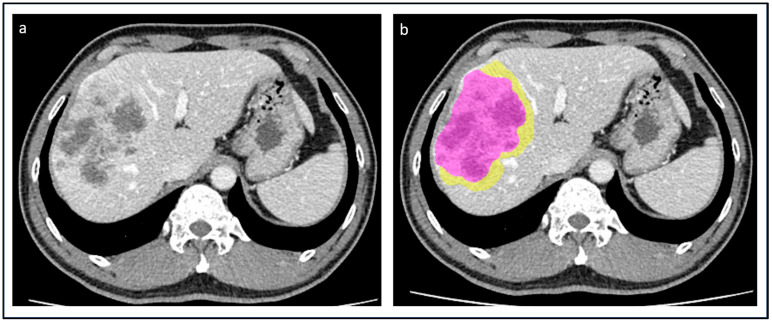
Patient with a mass-forming ICC of the right liver. (a) The tumor was identified on the portal phase of the CT; (b) manual segmentation of the tumor (violet area) and automatic segmentation of a 5 mm rim of peritumoral tissue (yellow area) were performed.
